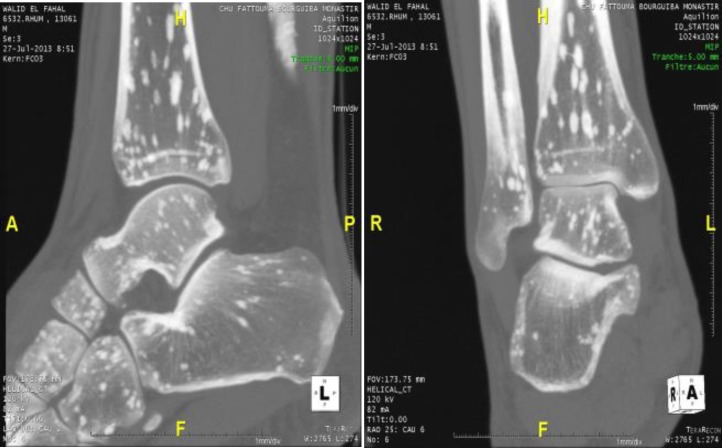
Computed tomography of the ankle: anteroposterior and lateral radiographs of the ankle. Sclerotic foci of variable size appear in the tibia and calcaneum
